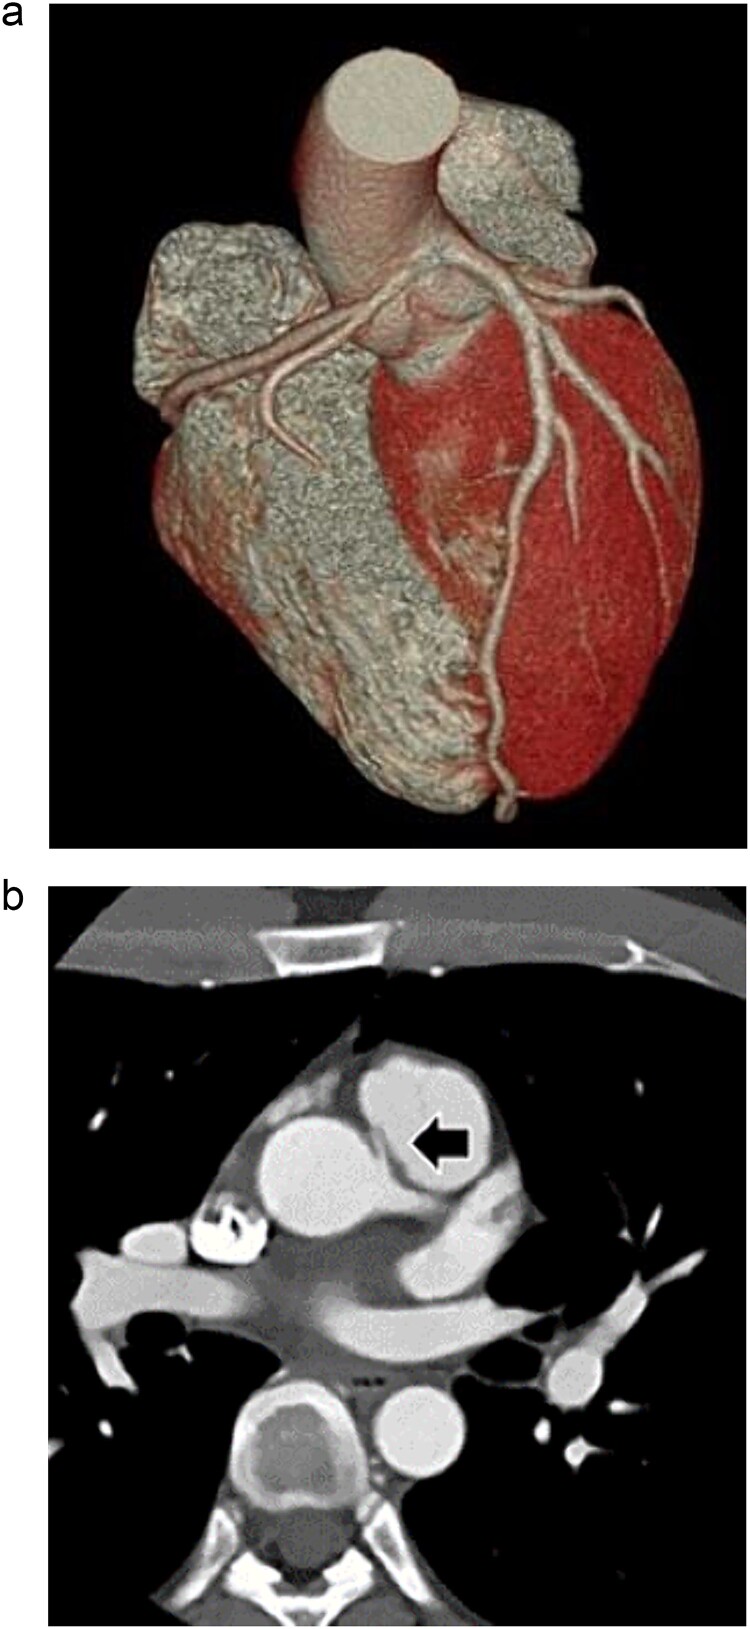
Preoperative three-dimensional CT, and enhanced CT shows AAORCA (a), and the right coronary artery (arrow) ran between the ascending aorta and the main pulmonary artery (b).
